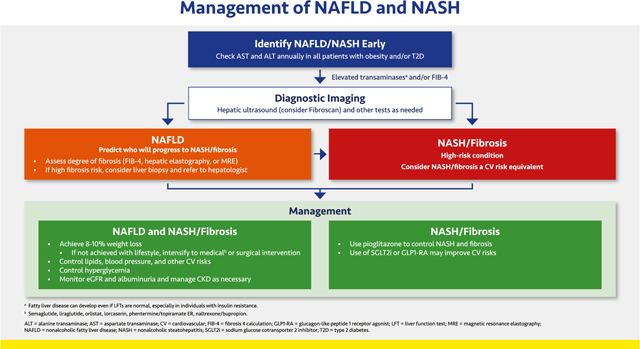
An abnormal ALT, AST, or FIB-4 may be followed up with ultrasound or MRI to assess hepatic fat content, and either Fibroscan or magnetic resonance elastography (MRE) used to determine the degree of hepatic fibrosis. Liver biopsy is the gold standard means of determining presence of NASH and is also useful for identifying other diseases that may cause or contribute to liver damage. The clinician should also consider and evaluate for other (or additional) potential etiologies of hepatic disease, including infectious hepatitis, hemochromatosis, and drug-related hepatotoxicity. Patients with or at high risk for fibrosis should be referred to a hepatologist.^146,147,150^
